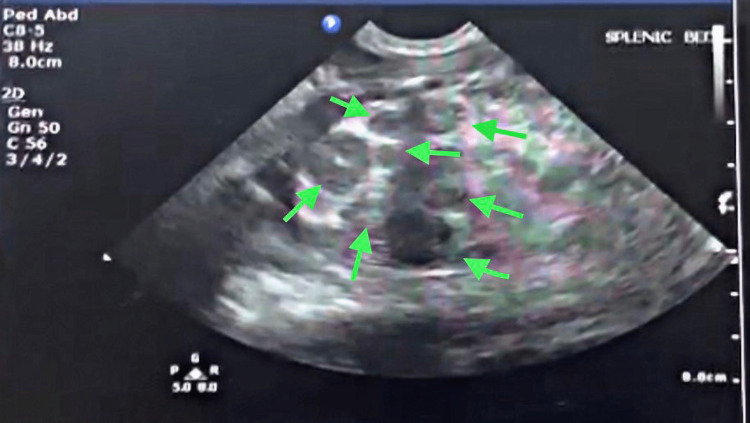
Abdominal ultrasound imaging of the left upper quadrant showing polysplenia.
